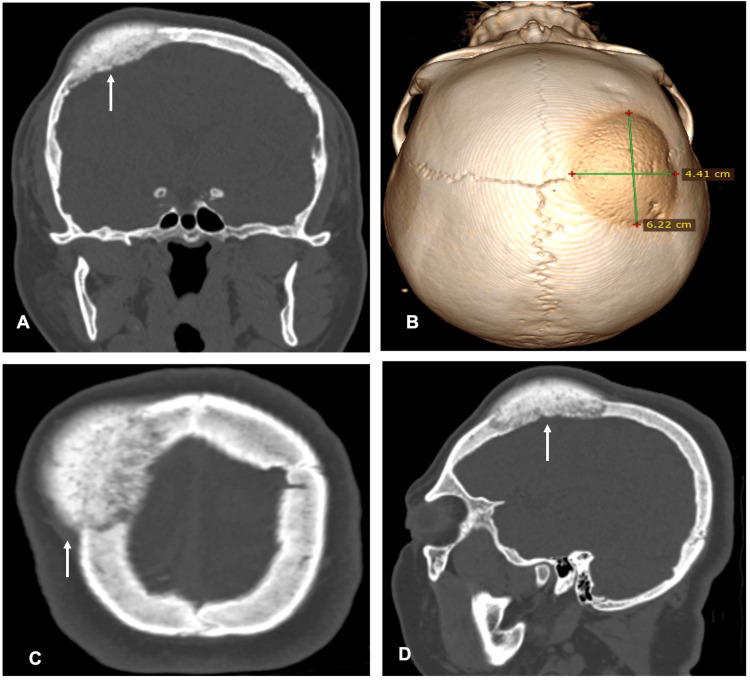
Cerebral computed tomography showing an osteoblastic lesion in the left frontoparietal region, with cortical thickening protruding beyond the margins of the adjacent normal bone. A. Coronal view. B. A 3D reconstruction from cerebral tomography, with measurements performed. C. Axial view. D. Left sagittal view.
